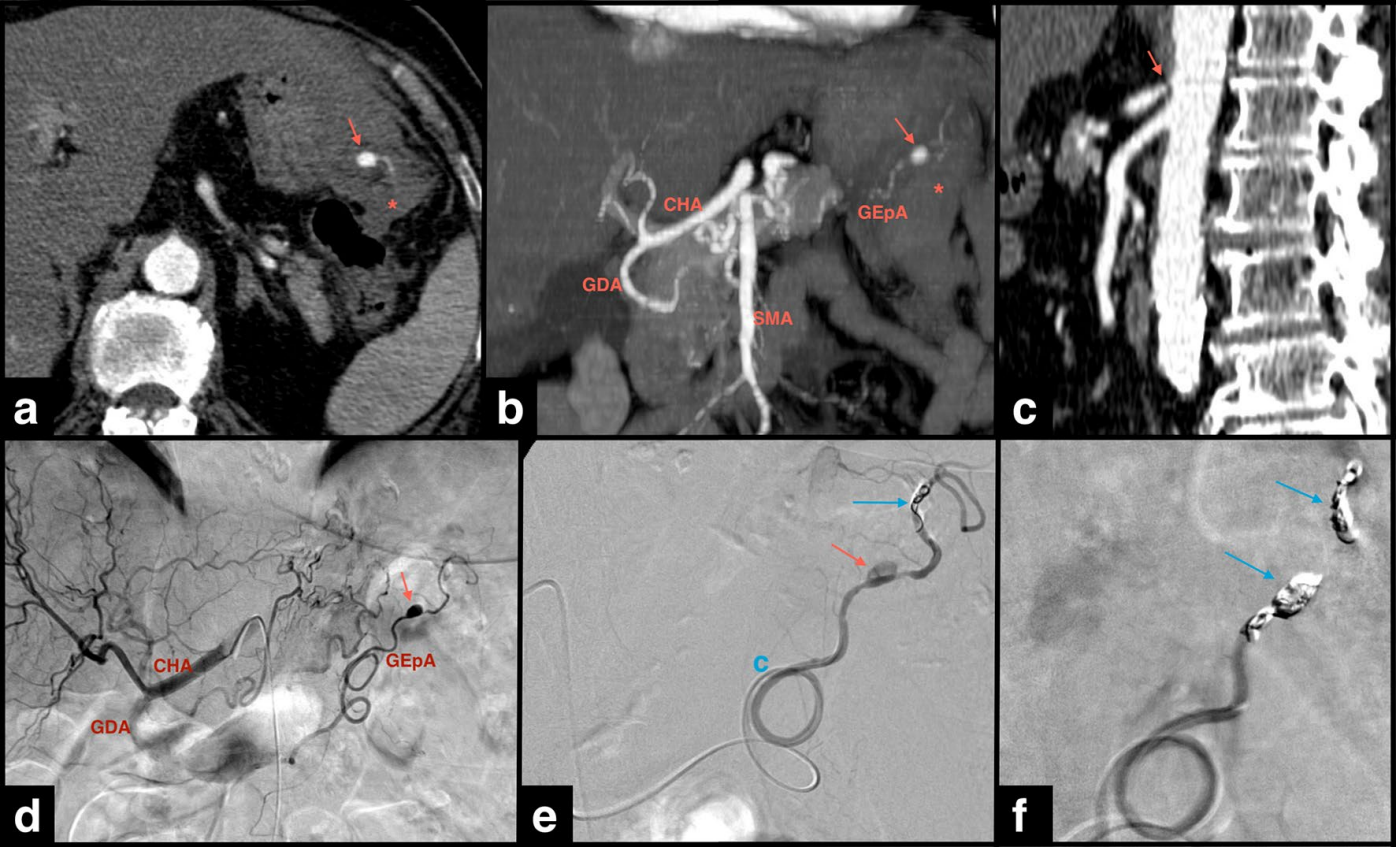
Ruptured aneurysm of the left gastroepiploic artery (GEpA) in a patient with celiac artery stenosis. Axial (a) and coronal MIP reconstruction (b) CTA images show the opacified aneurysm (arrows in a, b) with associated hemorrhage (*). Sagittal CTA image (c) shows the celiac artery compression by the median arcuate ligament (arrow). Arterial angiography images (d-f) show the GEpA aneurysm (red arrows). Both artery and aneurysm were embolized with microcoils (blue arrows), after selective catheterization with a coaxial microcatheter (c in image e). Post-embolization angiogram (f) shows no residual aneurysm opacification or bleeding. CHA, common hepatic artery; CTA, computed tomography angiography; GDA, gastroduodenal artery; MIP, maximum intensity projection; SMA, superior mesenteric artery
